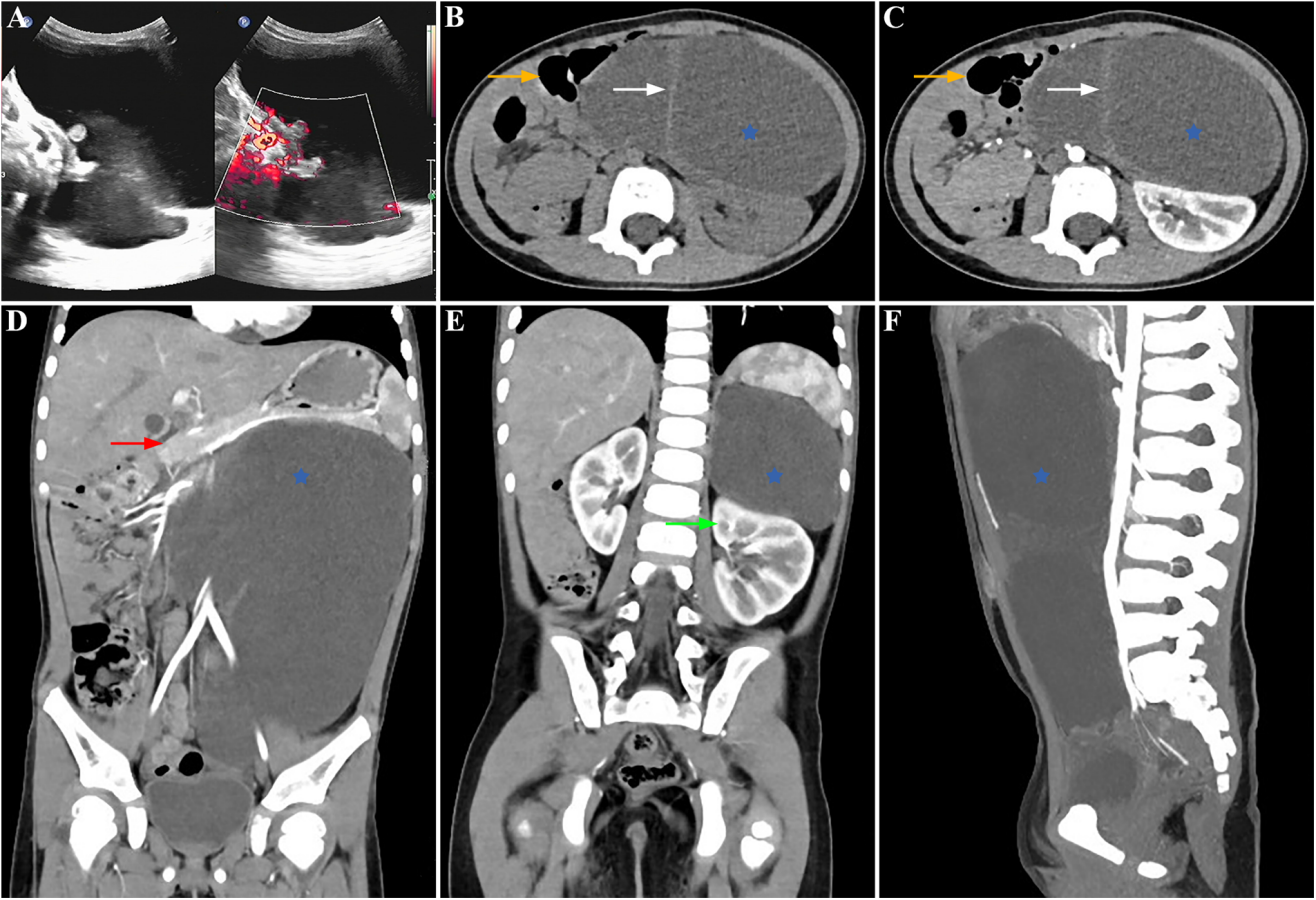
Preoperative findings: the lesion showed anechoic cysts with no obvious blood flow signal in the cyst wall in the left abdomen on an ultrasonogram (A). Contrast-enhanced abdominal-pelvic CT scanning showed a huge, slightly low-density lesion (blue star) with some septations (white arrow) in the retroperitoneum, which compressed the neighboring organs, pushing the left kidney downward (green arrow), pancreas upward (red arrow), and intestine rightward (orange arrow) (B,C: axial view, D,E: coronal view, F: sagittal view).
